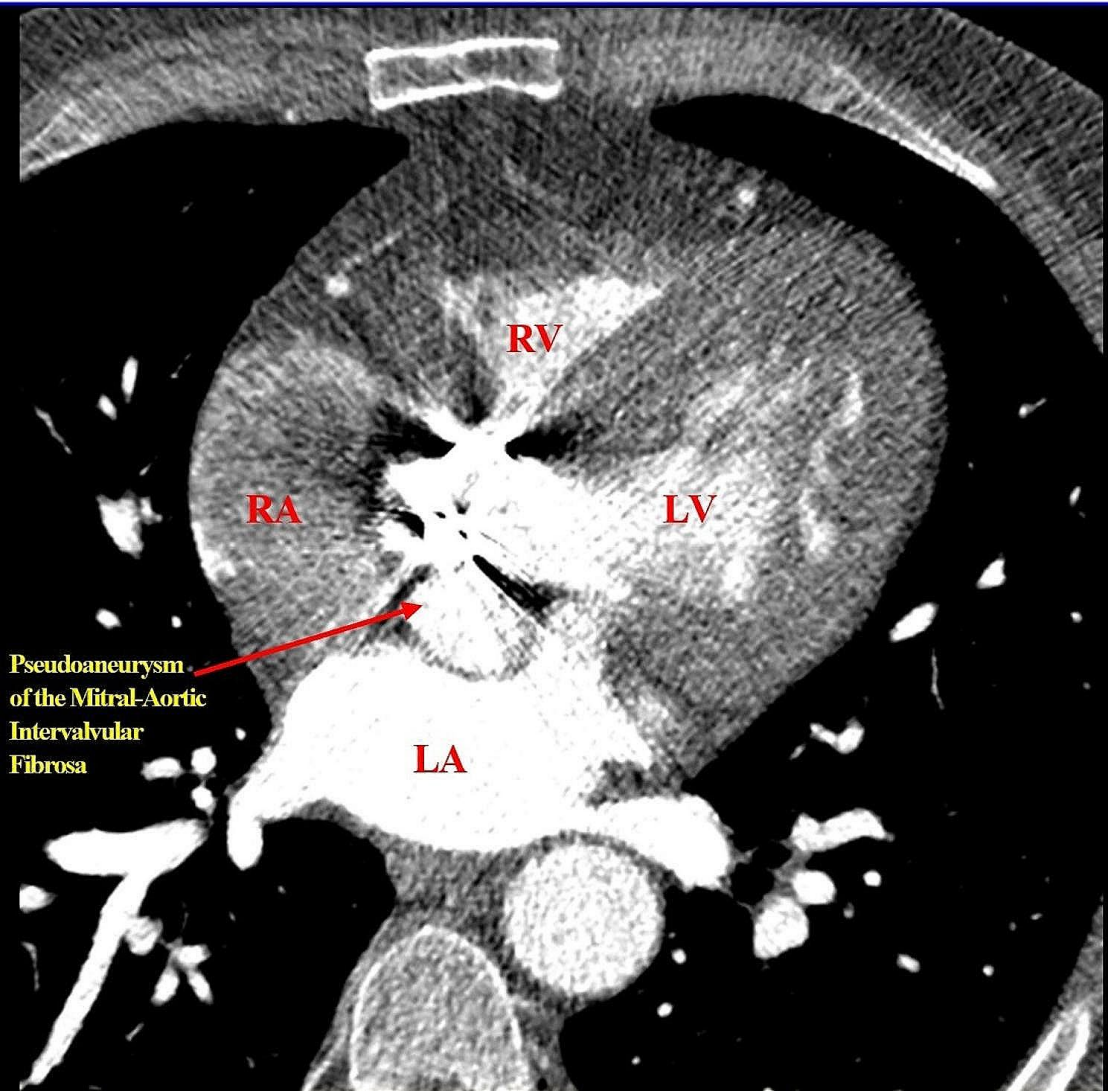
Contrast-enhanced computed tomography scan showing the PMAIF (arrow) in the axial plane, LV: left ventricle, RV: right ventricle, RA: right atrium, LA: left atrium
